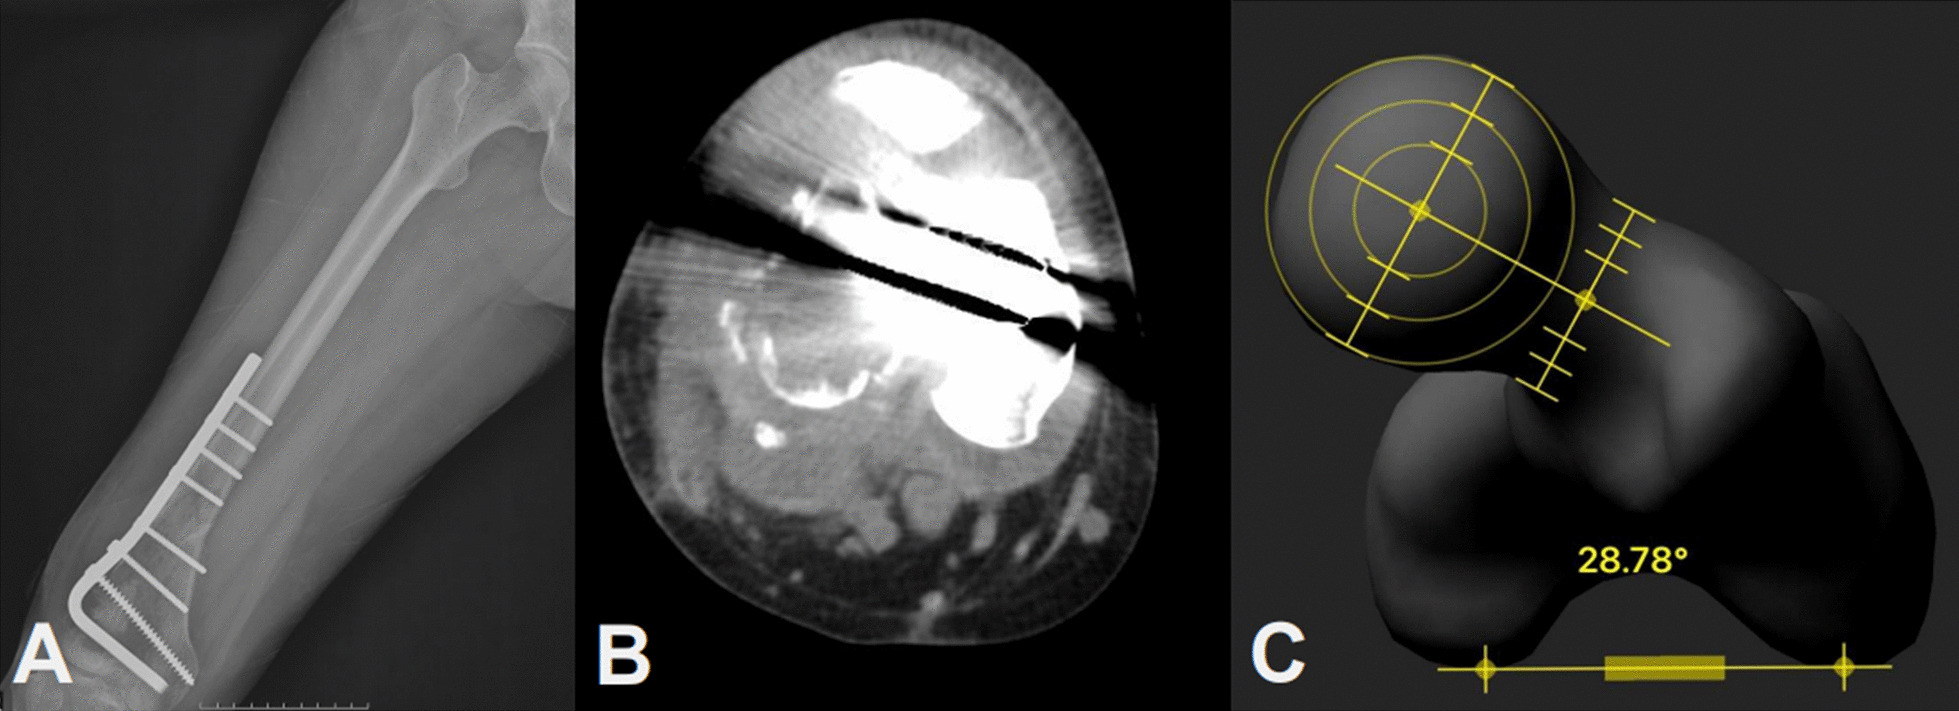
A 52-year-old female patient who underwent operation for a distal femur fracture. A Postoperative simple radiograph. B Rendering noise in the computed tomography (CT) image. C A three-dimensional (3D) model from the conventional femur anteroposterior (AP) and lateral (LAT) radiographs generated by the mobile application
